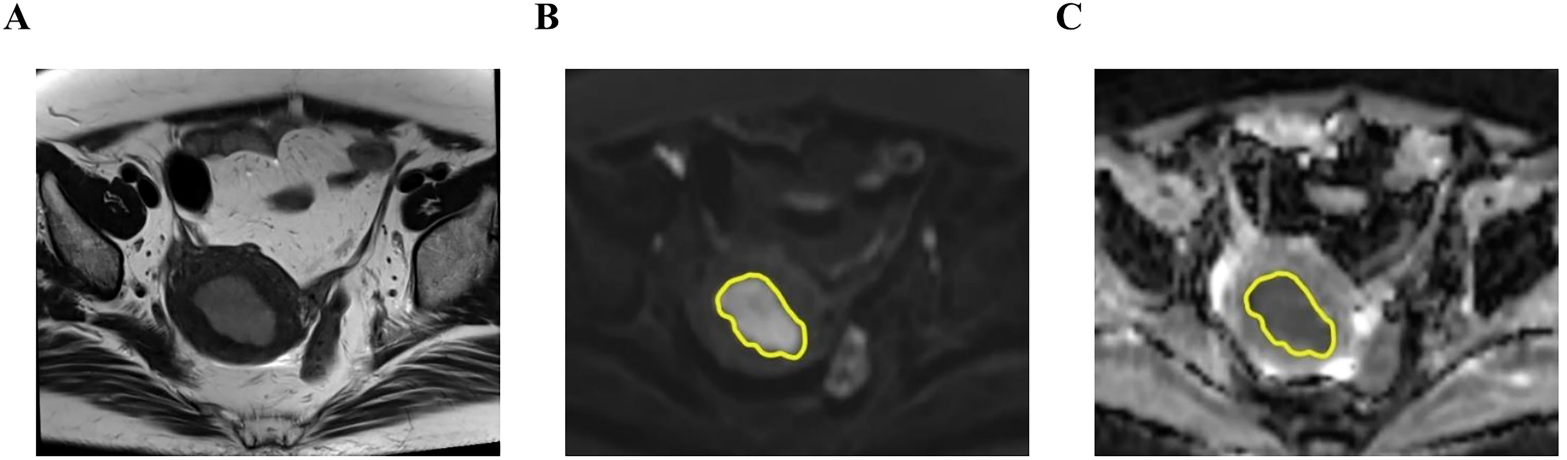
A 53-year-old woman with p53 abnormal endometrial cancer (endometrioid carcinoma, G3, stage IICm_p53abn_). The tumor shows slight hyperintensity on axial T2-weighted imaging (T2WI) (A), hyperintensity on axial diffusion-weighted imaging (DWI) (b=1000s/mm^2^) (B), and hypointensity on apparent diffusion coefficient (ADC) map (C). The region of interest (ROI) was drawn along the contour of tumor on DWI and subsequently transferred to the corresponding ADC maps through an automated process (yellow in B, C).
